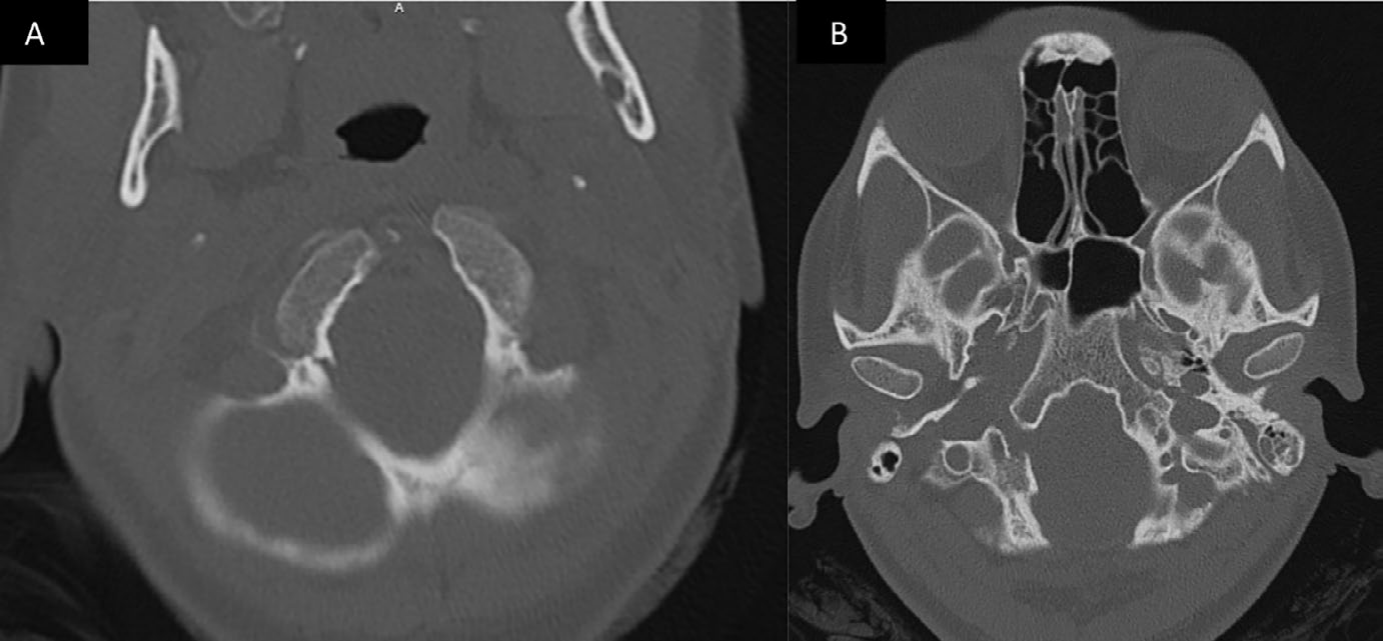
A and B non‐contrast CT maxillofacial (axial view) showing initial avulsion type fracture through right occipital condyle, and 3 months follow up CT showing narrowing of the hypoglossal canal due to callus formation.
